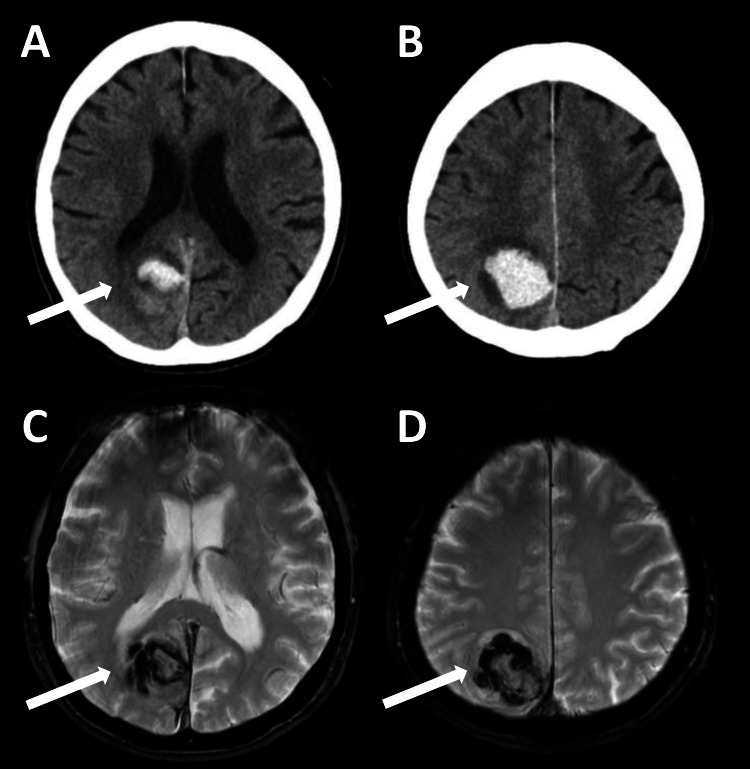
CT and MRI of the brain upon presentation. CT (A, B) and T2*-weighted MRI (C, D) of the brain obtained upon presentation demonstrating a subcortical hemorrhage in the right occipitoparietal region, accompanied by surrounding edema.
